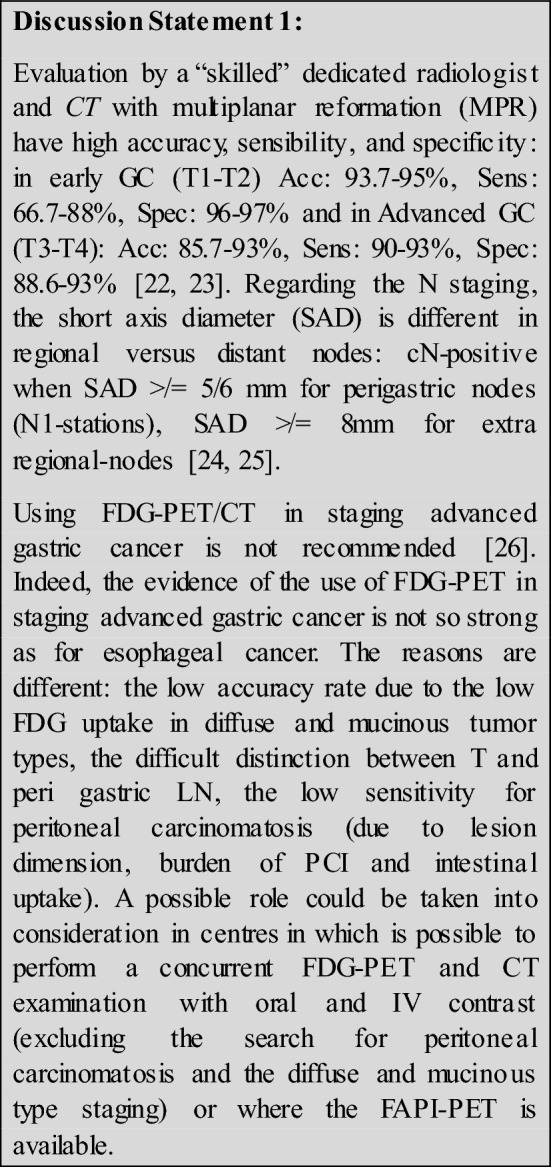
2.How do you stage cancer regression to first-line treatment?CT scan (using % of reduction not strictly limited to RECIST) (41%)b. CT scan D Max (0%)Texture Analysis (0%)CT using RECIST (59%)
